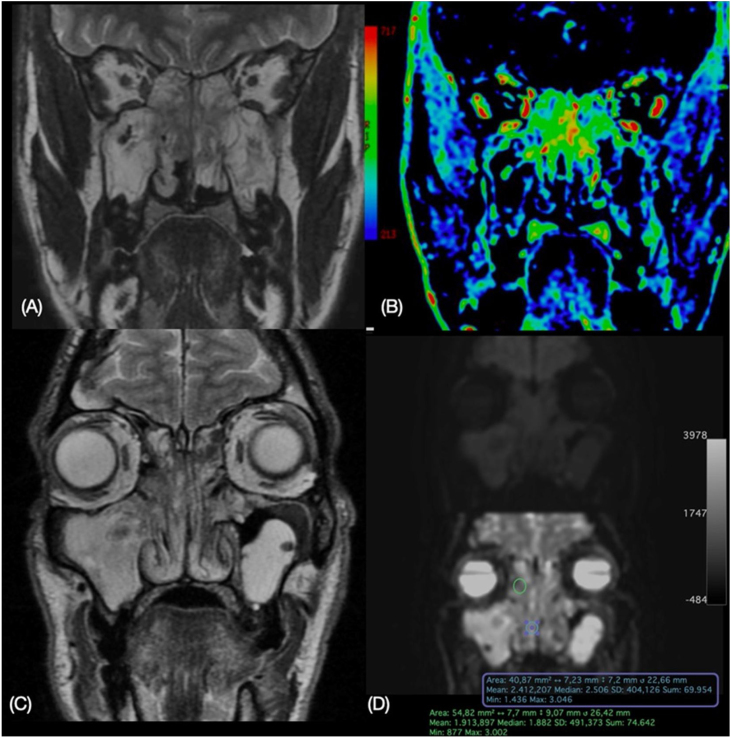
(A) T2-weighted image and (B) perfusion (PWI) color map shows increased perfusion areas in the central portion than in the peripheral portion, (C) T2-weighted image and (D) diffusion (DWI) and ADC map with values calculated by positioning the ROI in the central portion – green circle – and in the peripheral portion — blue circle.
